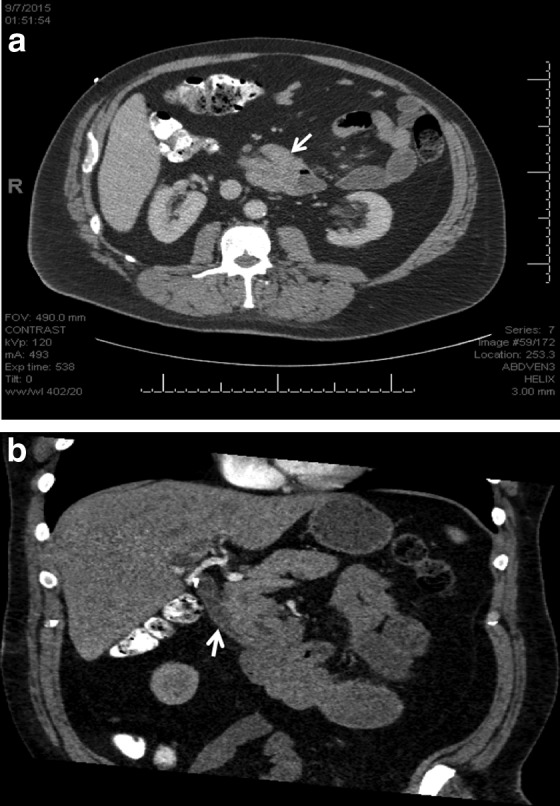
(a, b) Axial and coronal CT scan slices showing invagination of proximal portion of duodenum into distal (white arrows). CT, computed tomography.
